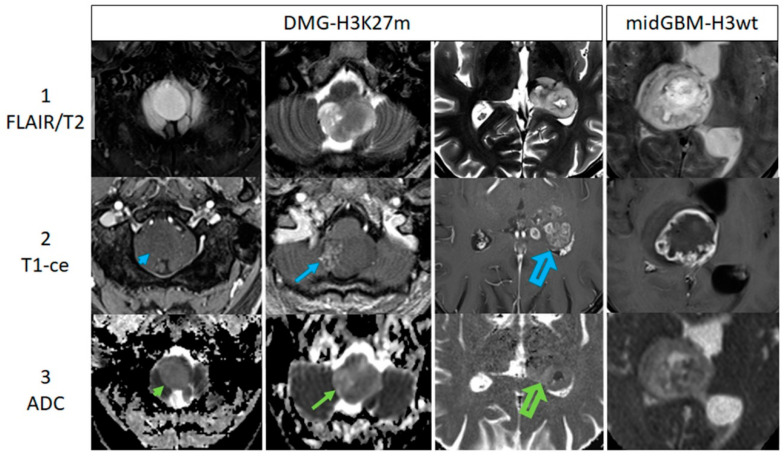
This figure displays examples of MR imaging findings including DWI/ADC. The cases of four patients with midline gliomas are shown in columns from left to right (row 1 presenting FLAIR/T2w-images, row 2 representing ce-T1w images, and row 3 showing ADC maps). The first three columns present DMGs with increasing contrast enhancement from left to right: no enhancement (blue arrowhead), low enhancement (blue arrow) and strong enhancement (blue open arrow). The fourth column shows a case of a midline glioblastoma (midGBM-H3wt). Corresponding ADC-maps are shown in row 3, indicating the variance of inhomogeneity (green arrowhead/arrows pointing at the tumor).
